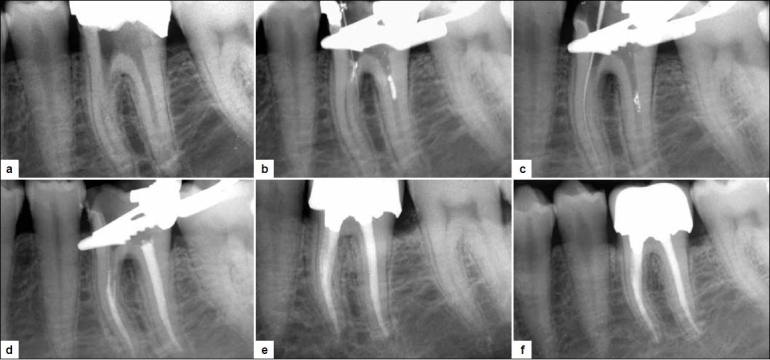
a) Pre-operative radiograph showing radio-opaque filling material coronally with loss of continuity of lamina duer and radiolucency at the mesial furcation with widening of periodontal ligament space in mesial root apex. b) Detection of strip perforation with no. 08 K-file in the mesiobuccal canal. c) Patency of the mesial canals established. d) Mineral trioxide aggregate obturation in mesiobuccal canal till the orifice; ML and Distal canals were obturated simultaneously. e) On follow up at 6 months, furcal bone formation is evident. f) In the 2-year follow-up radiograph, osseous regeneration is observed at the apex and furcation; normal periodontal ligament space is observed
